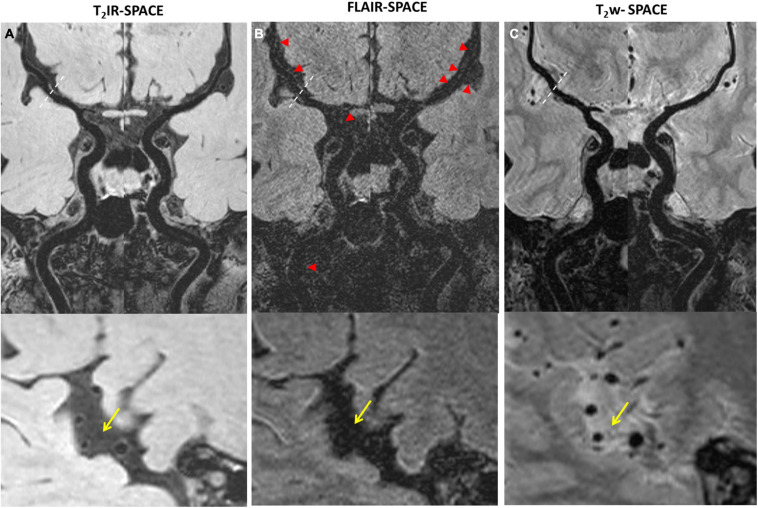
Comparison among (A) T_2_IR-SPACE, (B) FLAIR-SPACE, and (C) T_2_w-SPACE images in a healthy 60-year-old volunteer. Curved multi-planar reconstruction images of both the left and right sides, starting from the internal carotid artery (ICA) and continuing up to the M2 segment of the middle cerebral artery (MCA), were generated for visual comparison. Bottom images show the zoomed-in image to better show the inner/outer vessel wall for each sequence, CSF area are labeled by the arrows. (A) The vessel walls are clearly visualized at all segments of intracranial arteries on T_2_IR-SPACE. (B) The SNR is low on FLAIR-SPACE, and most of the intracranial vessel wall cannot be delineated (indicated by the red arrowheads). (C) For T_2_w-SPACE, delineating the outer boundary of the intracranial artery wall is challenging.
